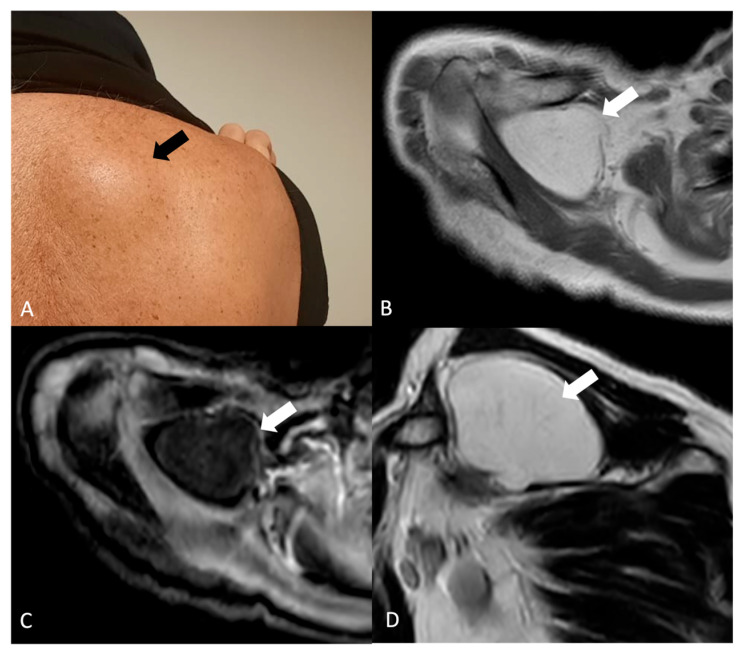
(A) Right shoulder bump of a 67-year-old male patient (black arrow). (B) Axial T1-weighted, (C) axial T1-weighted-fs and (D) sagittal T2w show a fat-containing (white arrow) lesion. No internal septations or nodular components are observed. The imaging features and typical superficial location are conclusive for a benign lipoma.
